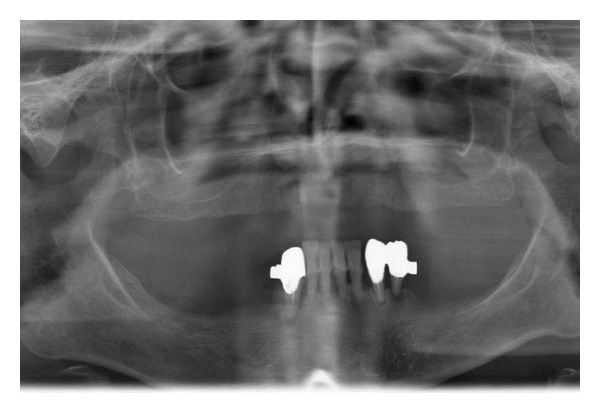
Panoramic X-ray with periodontal teeth.
